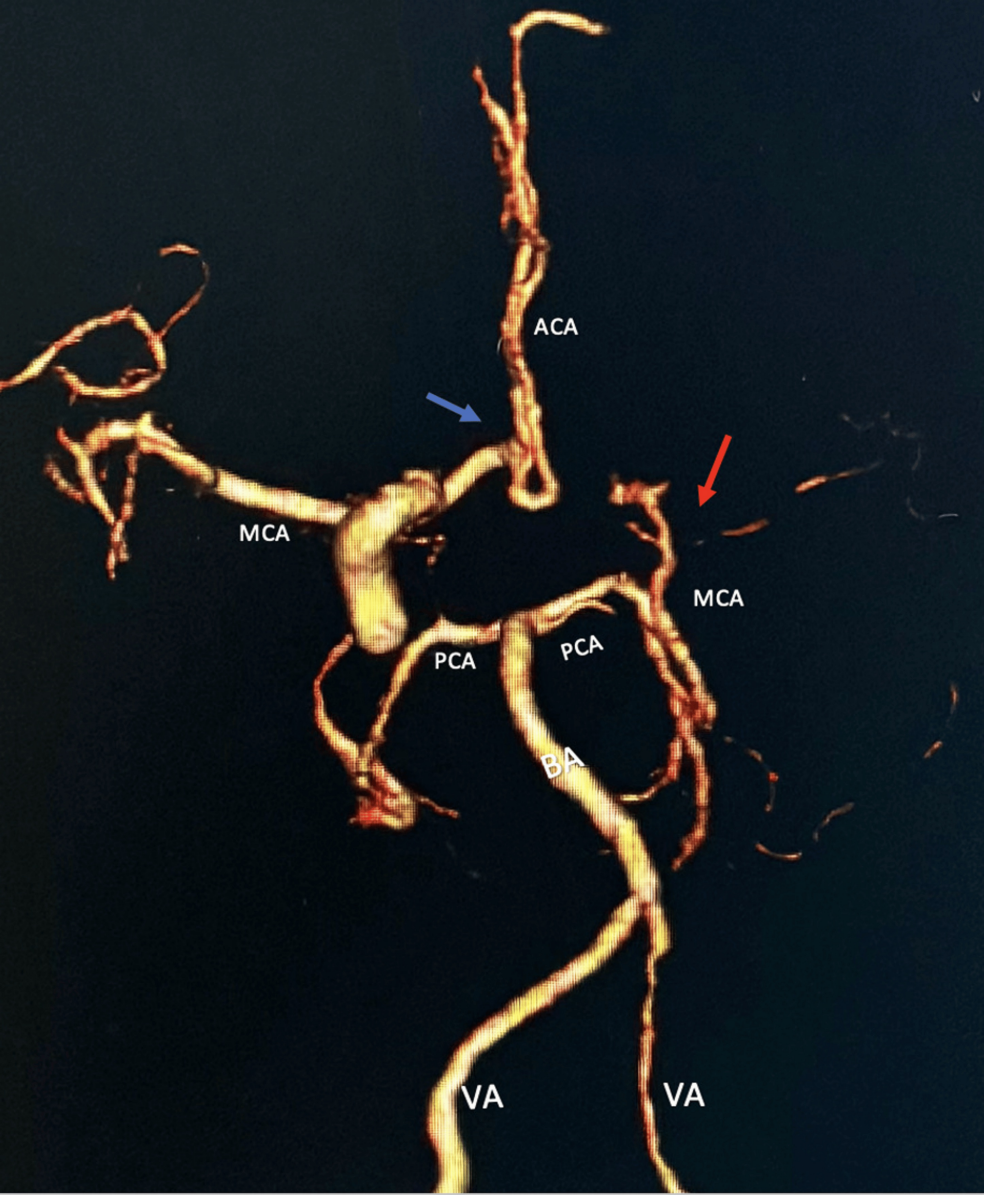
CTA of head showing a complete occlusion of the left M1 segment (red arrow), with minimal distant M2 and M3 branches visualized. The right MCA branches are unremarkable with mild narrowing of the proximal right A1 segment (blue arrow), and left ACA is poorly visualized. VA, vertebral artery; BA, basilar artery; PCA, posterior cerebral artery; MCA, middle cerebral artery; M1, first segment of MCA; M2, second segment of MCA; M3, third segment of MCA; ACA, anterior cerebral artery; A1, first segment of ACA; CTA: computed tomography angiography
